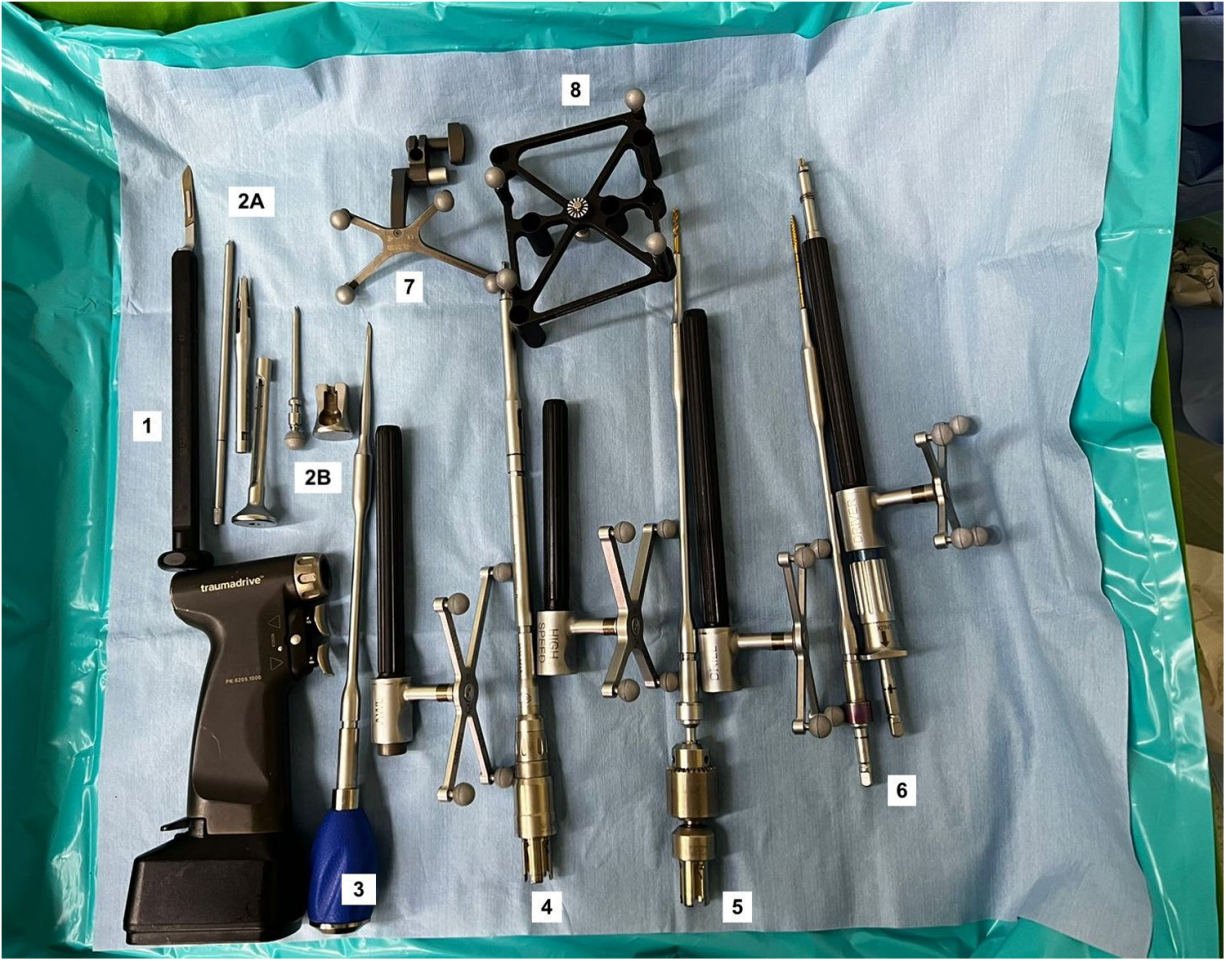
Tools used in the procedure. (1) Scalpel, (2A) Low profile quattro spike, bine clamp, and guide post, (2B) Surveillance marker, (3) Awl and array, (4) Drill and array, (5) High burr and array, (6) Tapper and array, (7) Dynamic Reference Base, (8) Intraoperative Cone-beam Tomography.
